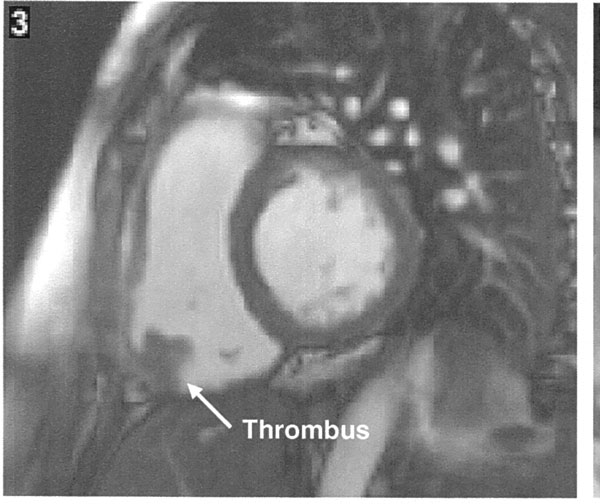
Cardiac magnetic resonance imaging showing the thrombus attached to the right ventricle.
